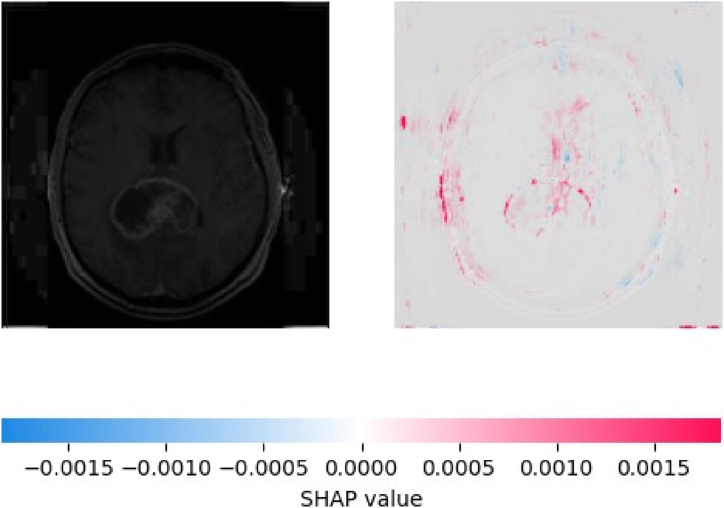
MRI image (left) and its SHAP explanation (right), highlighting regions indicating the tumor prediction.
